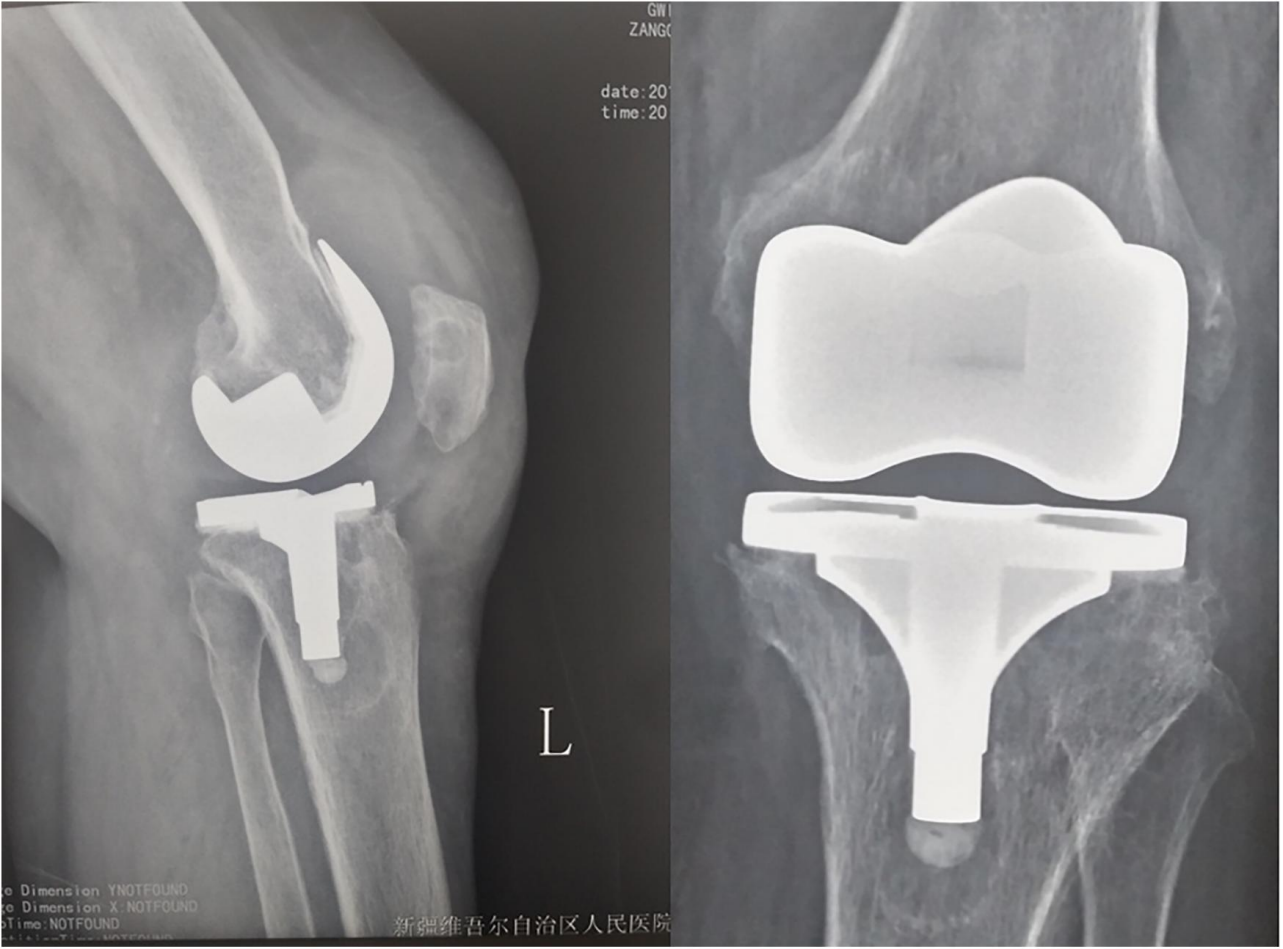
Pre-operation x-ray showed prosthesis loosing.
